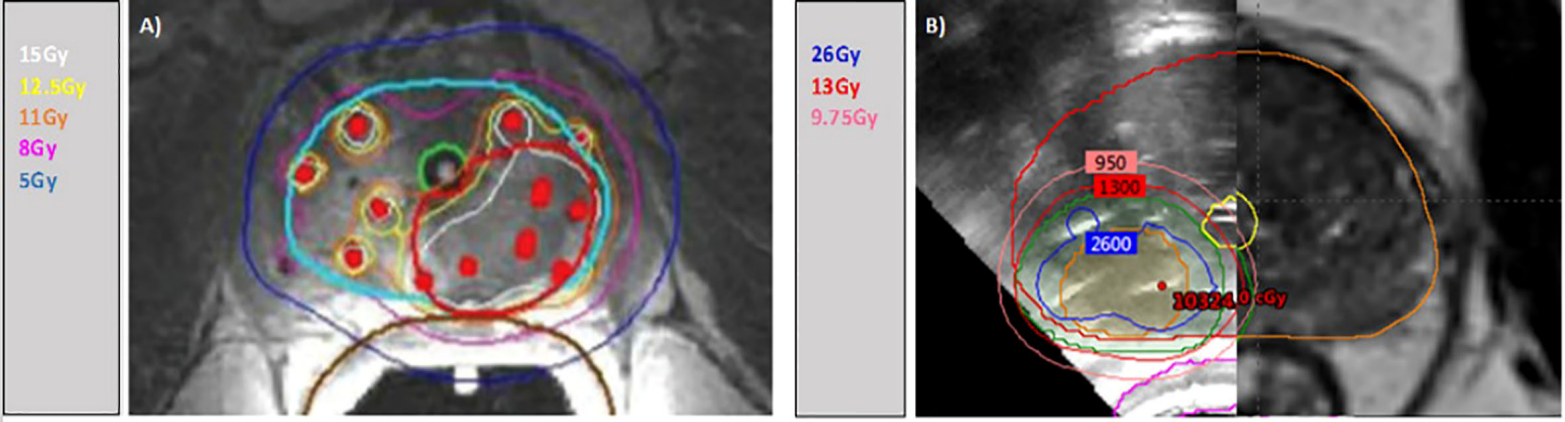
Isodose distributions for case examples. (A) ibBT with MRI-only guidance. Contoured in light blue the CTVwp, in red the PTV (GTV). Isodose lines: white 15Gy (135%), yellow 12.5Gy (114%), orange 11Gy (100%), pink 8Gy (75%) and dark blue 5Gy (50%). (B) fBT with TRUS/MRI guidance. Contoured in orange the GTV and green the PTV (GTV). Isodose lines: blue 26Gy (200%), red 13Gy (100%), pink 9.75Gy (75%).
